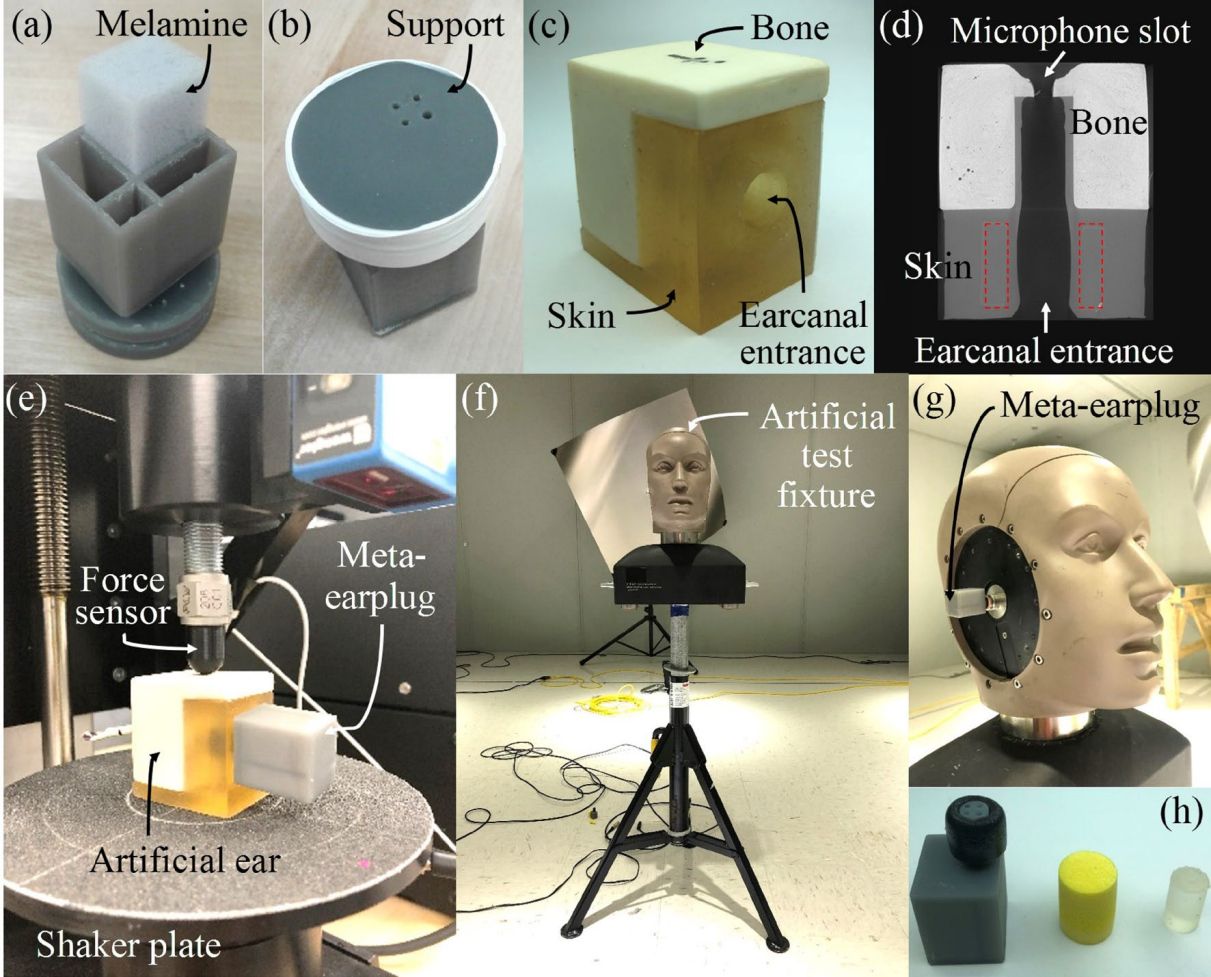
(a) Lateral and (b) medial view of the meta-earplug including a built-in support for impedance sensor measurement and showing the insertion of melamine foam in HR cavity before gluing the back-plate. (c) Artificial ear designed for occlusion effect measurements and (d) CT-scan performed in the horizontal plane showing the geometry of the artificial earcanal and its surrounding tissues (red dotted rectangles indicate the position of the cartilaginous ring surrounding the earcanal but difficult to distinguish from the skin tissue). (e) Experimental setup for occlusion effect assessment using a QMA facility. (f) Experimental setup for measuring the insertion loss of earplugs using an artificial test fixture placed in a reverberant room. (g) Meta-earplug inserted in the earcanal of the artificial test fixture. (h) Size comparison between the meta-earplug (left), the foam (middle) and the silicone (right) earplugs used in the current work.
